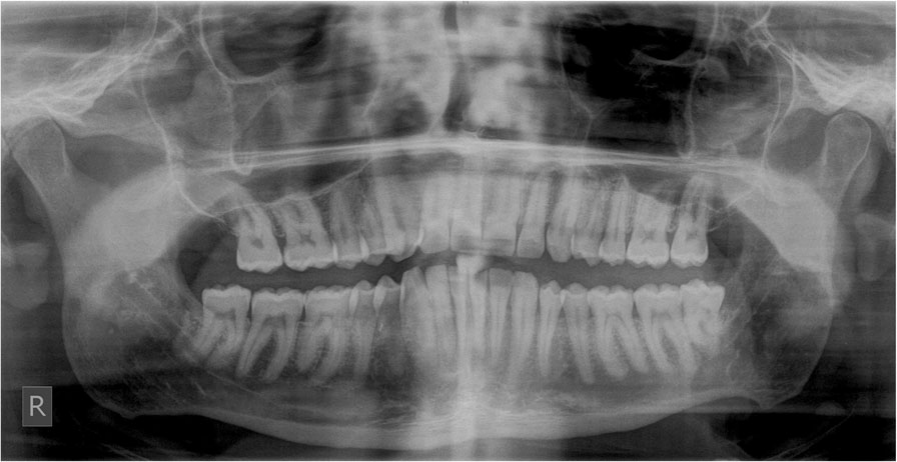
The orthopantomography (OPG) of our patient revealed no significant radiological findings
